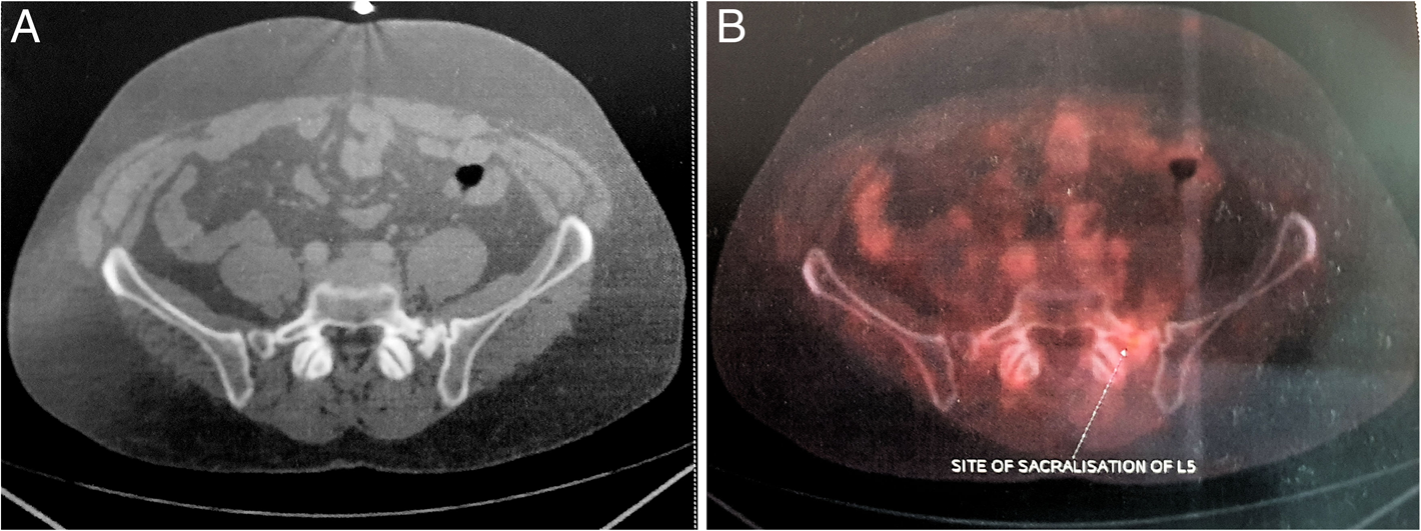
Follow-up FDG-PET/CT in 2018. a Sclerotic lesion in the left sacral ala. b Uptake noted at the site of sacralization (SUV 4)
